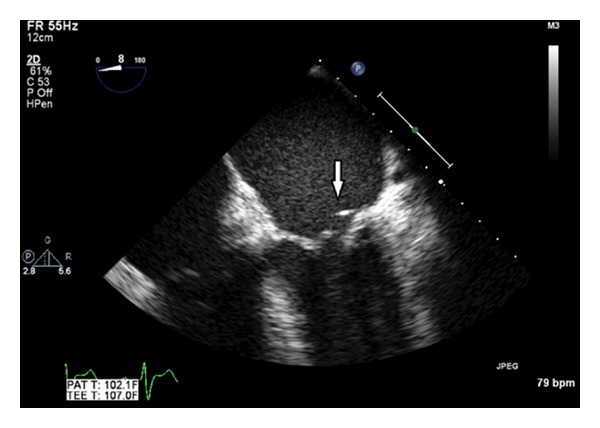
Transesophageal echocardiogram. A small vegetation (white arrow) on the mitral leaflet at the site of mitral valve repair.
